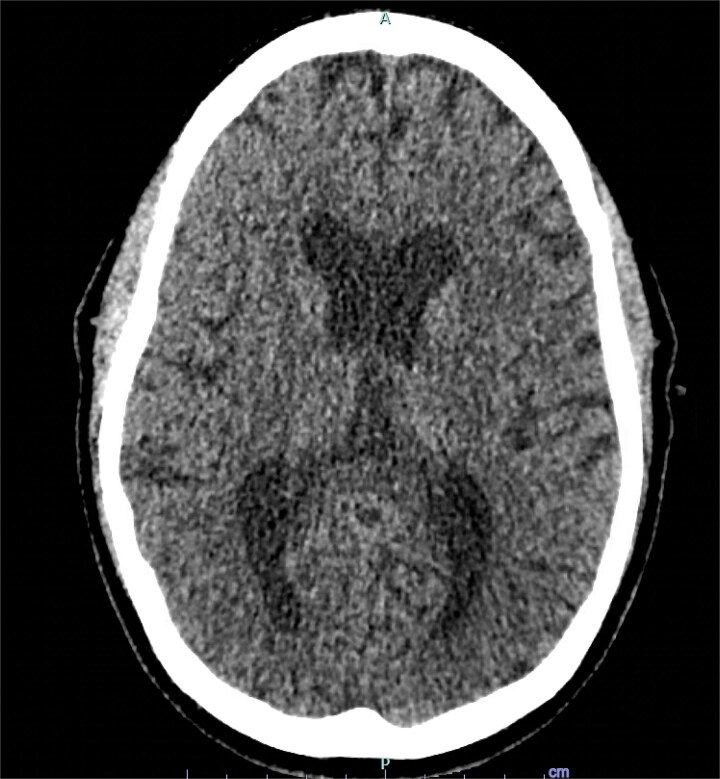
Computed-tomography (CT) of the head from 04/03/2025 demonstrating no evidence of hemorrhage, infarct, or large-vessel occlusion.
